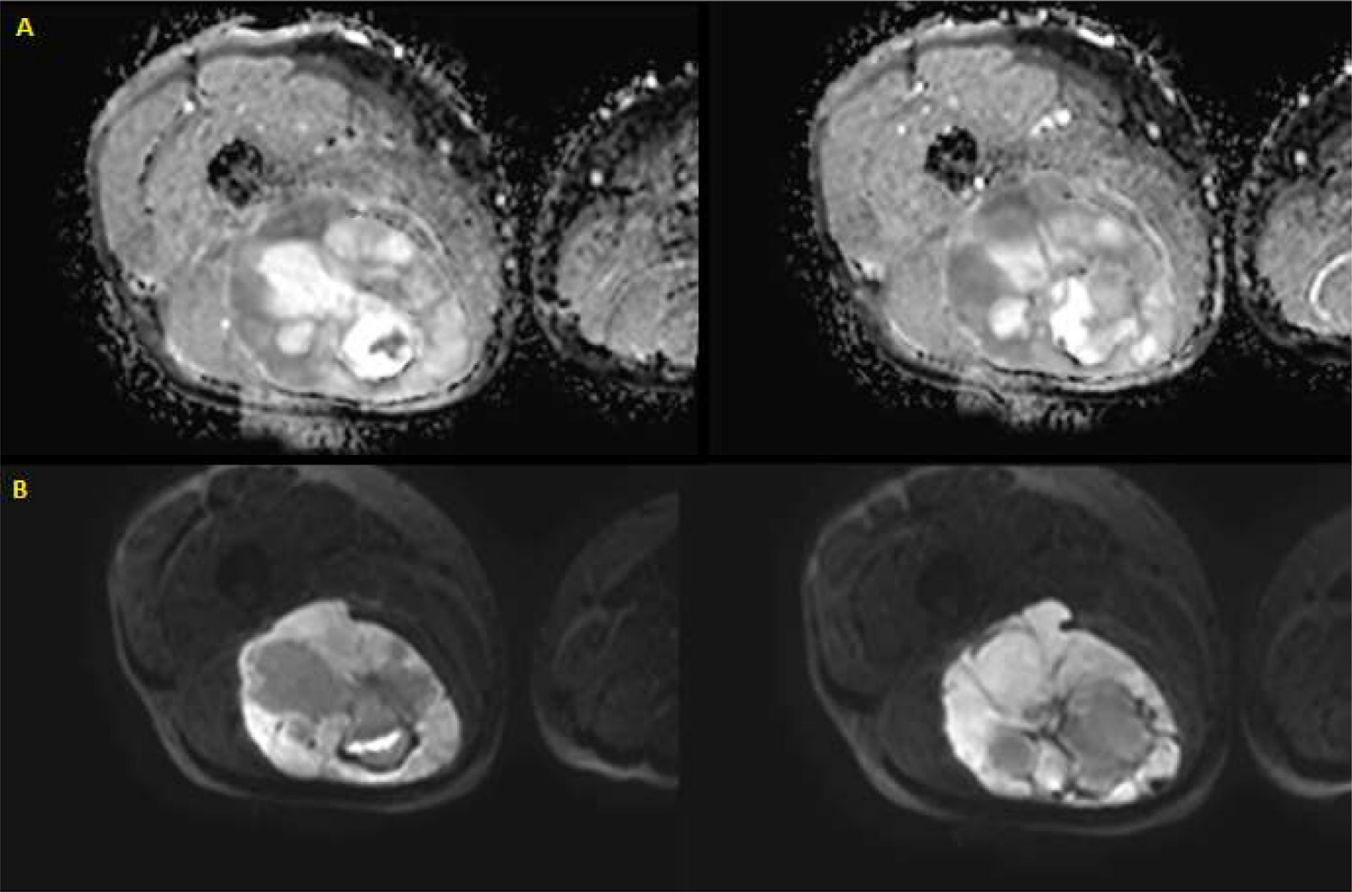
MRI of the posterior region of the right thigh: (A) in the axial section in DWI (diffusion-weighted) at the major level of B-value (s/mm^2^); (B) in the axial section ADC-map; those 2 couple of images are at different levels of the lesion. There were different areas of high cellularity, narrowing in diffusion and confirming the suspicion of malignancy.
